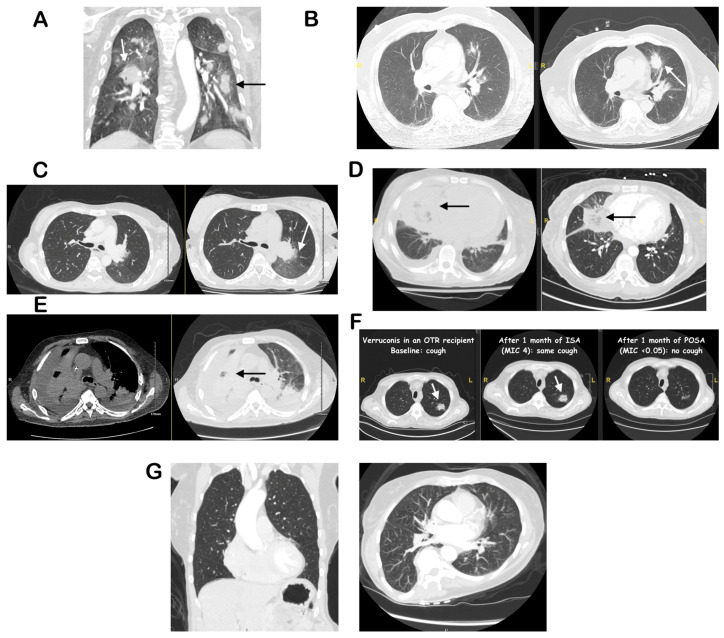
Arrows indicate characteristic abnormal findings. (A): Multifocal consolidations in the setting of high-dose steroids, serum GM > assay cut-off (OD 8), autopsy showed angioinvasive septate narrow-angled hyphae, consistent with IPA. (B): Enlarging nodule with “halo sign” in a neutropenic patient with acute myeloid leukemia, positive serum bDG, BAL PCR and plasma mcfDNA positive for *Aspergillus fumigatus*. (C): Nodule with “halo sign” in a neutropenic patient with aplastic anemia, positive BAL Galactomannan and plasma mcfDNA positive for *Aspergillus flavus*/*oryzae*. (D): “Reverse Halo” sign with rapid progression off treatment, negative BAL and tissue biopsies. Plasma mcfDNA-NGS was positive for *Rhizopus delemar* and autopsy showed angioinvasive aseptate broad-angled hyphae consistent with mucormycosis. (E): Large consolidation with BAL growth of a *Syncephalastrum* species (not further speciated). (F): Multiple pulmonary nodules with BAL growth of *Verruconis galopava* (“black” mold), and response to different anti-mold agents (OTR: Organ transplant recipient, POSA: Posaconazole, ISA: Isavuconazole), correlating with its minimal inhibitory concentrations (MIC). (G): Normal chest CT.
